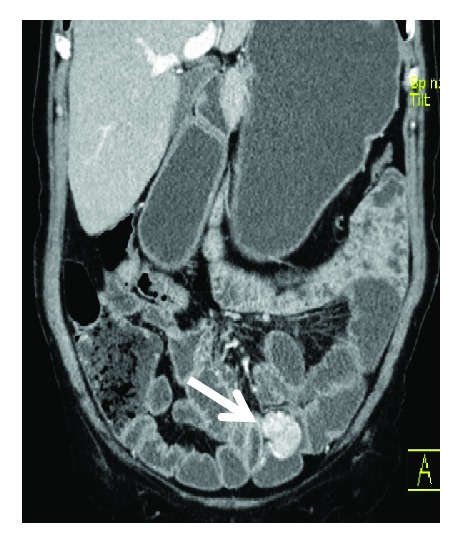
CT enterography—coronal CT image lesion compatible with GIST.
